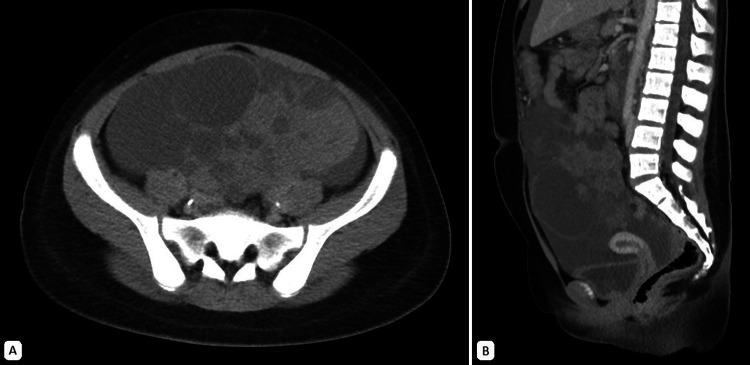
Abdominal CT scan, venous-phase. An extensive heterogeneous mass with lobulated borders and heterogeneous enhancement, likely of left adnexal origin, measuring 12.9 x 12 x 13.5 cm. It is associated with abundant free fluid in the abdominopelvic cavity, along with reticulations of the peritoneal fat planes. A. Axial plane B. Sagittal plane
